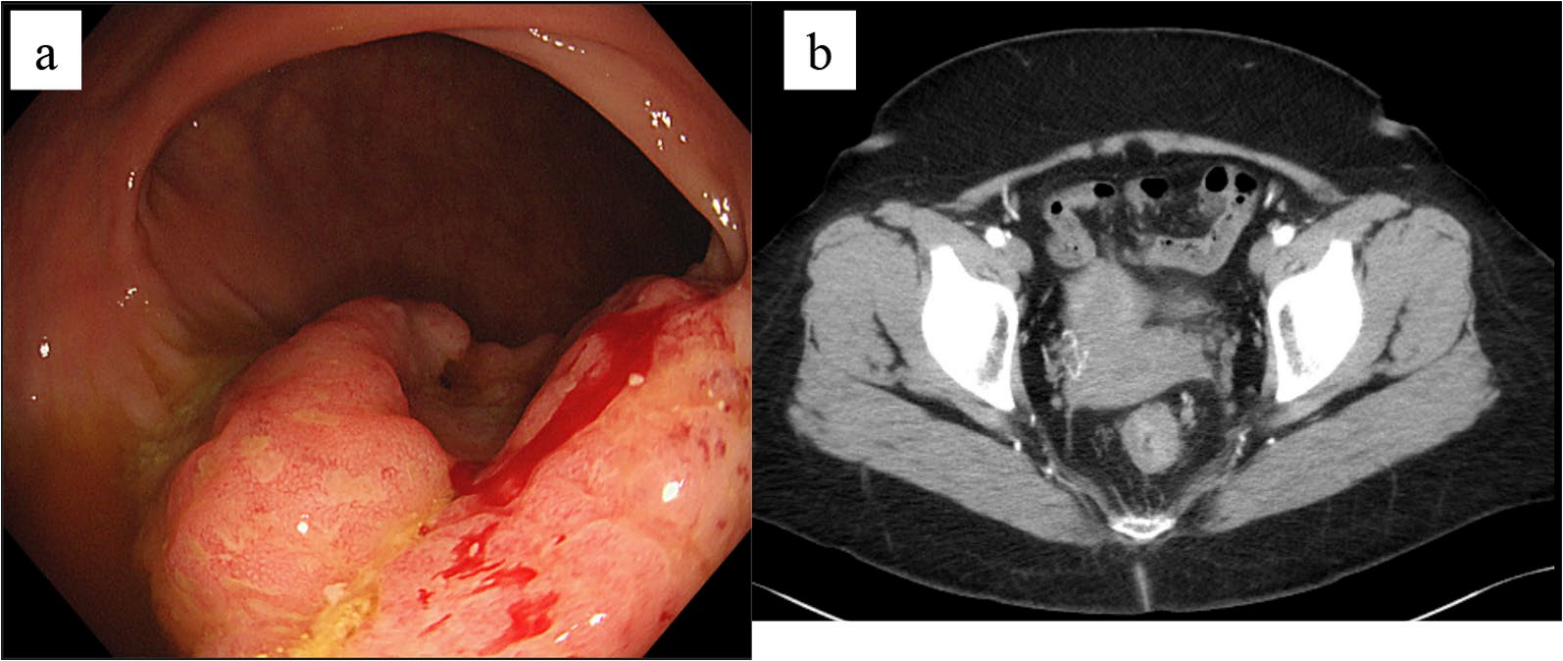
A Colonography revealed an advanced rectal cancer. B Computed tomography scan revealed a hypervascular irregular wall thickness in the rectum and swollen lymph node
